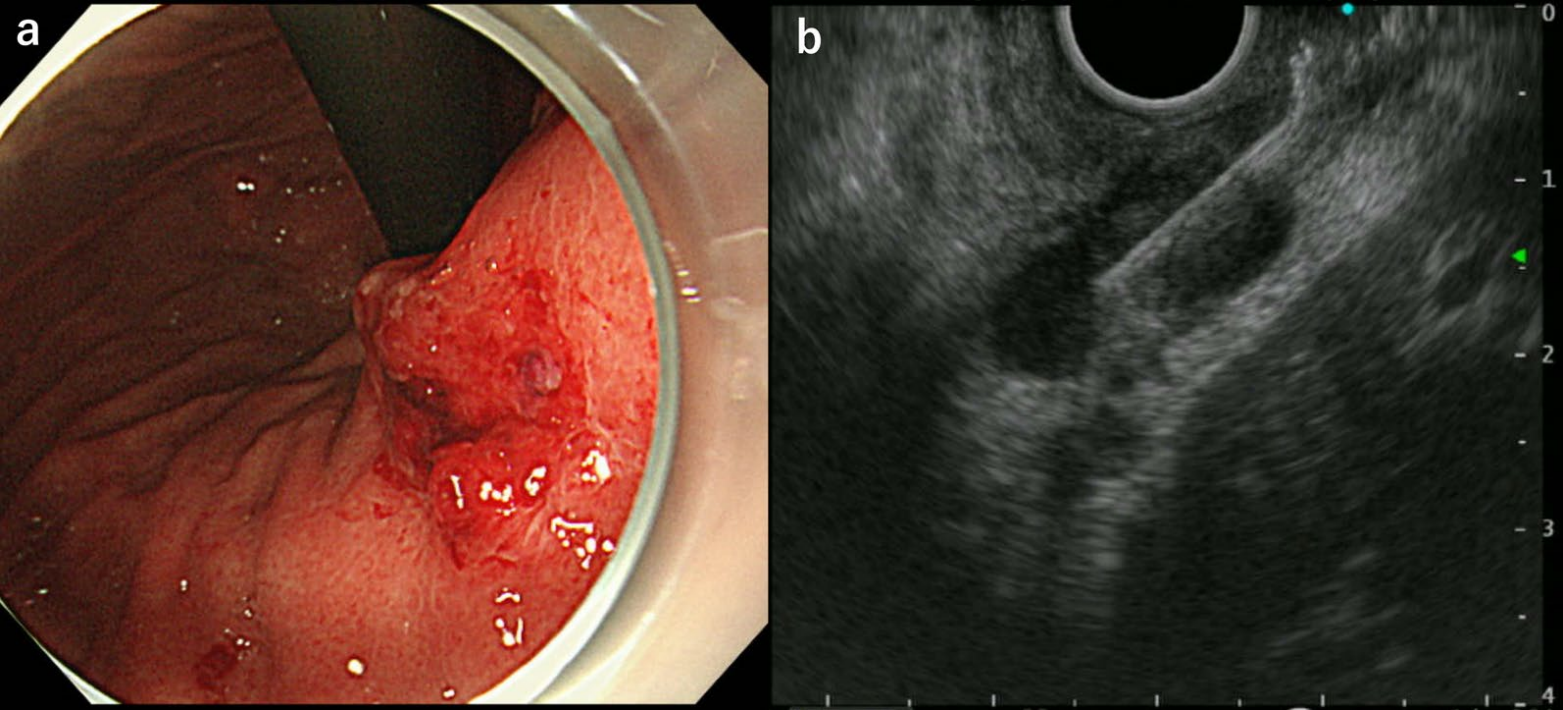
Images of the second gastrointestinal endoscopy and ultrasound endoscopy. a An ulcerative lesion is found on the lesser curvature of the upper body of the stomach. b Endoscopic ultrasound-guided fine-needle aspiration was performed on lymph nodes contiguous with a gastric ulcer
